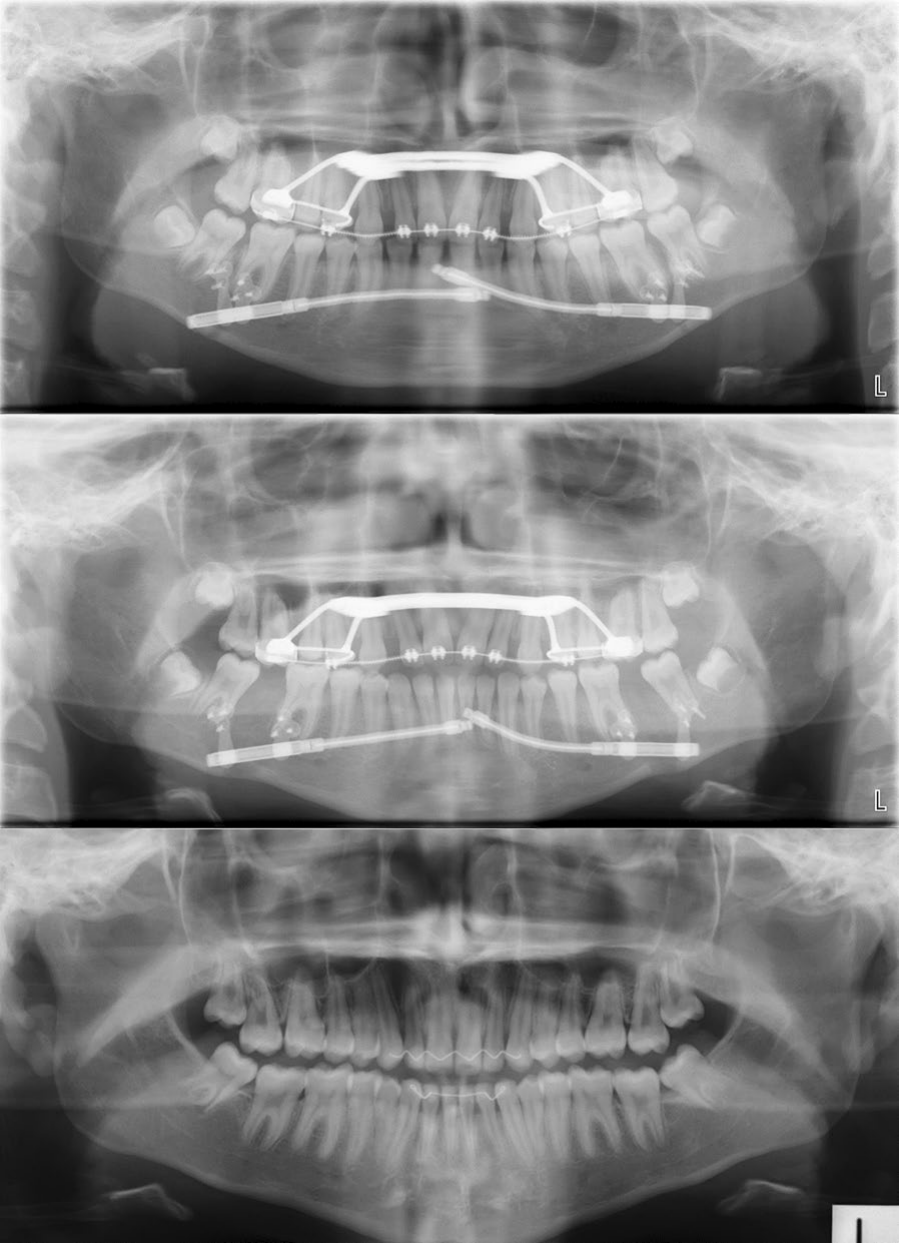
Orthopantomograms of subject #08 at T0, T1 and T2 showing the distractors, amount of distraction and consolidation of the mandibular body, respectively
